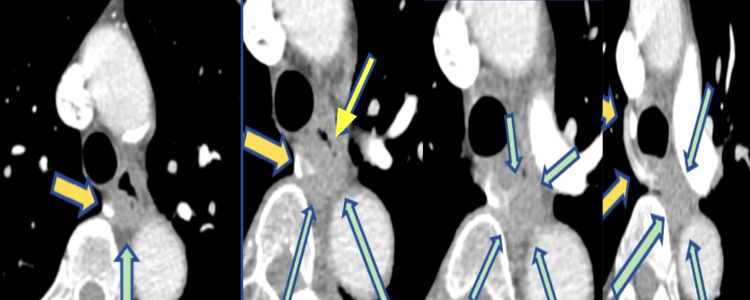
CT chest images Images show a 1.36 x 1.77 x 1.96 cm soft tissue mass along the esophagus's posterior wall (blue-green arrows) at the distal trachea and T5-T7 vertebrae level as a right apical pleural-based thick-walled soft tissue density. Yellow arrows show a hemizygos vein with a thickening of the wall.
